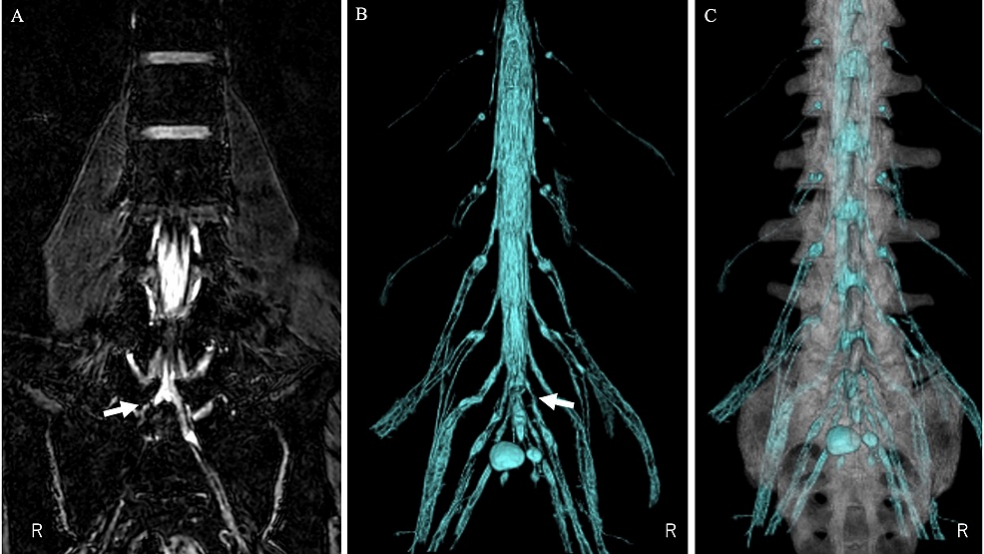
CT/MRI fusion image of case 2 The left image (A) shows the nerve root in the T2 FFE PROSET sequence. The S1 nerve root is disrupted by the herniation (arrow). The middle image (B) is a 3D constructed MRI image of the dural tube and the nerve roots in the T2 FFE PROSET sequence on the left image. The dural tube and nerve root are partially lost due to herniation at the arrow. The right image (C) is a CT/MRI fusion image of a 3D CT image and a 3D MRI image.
